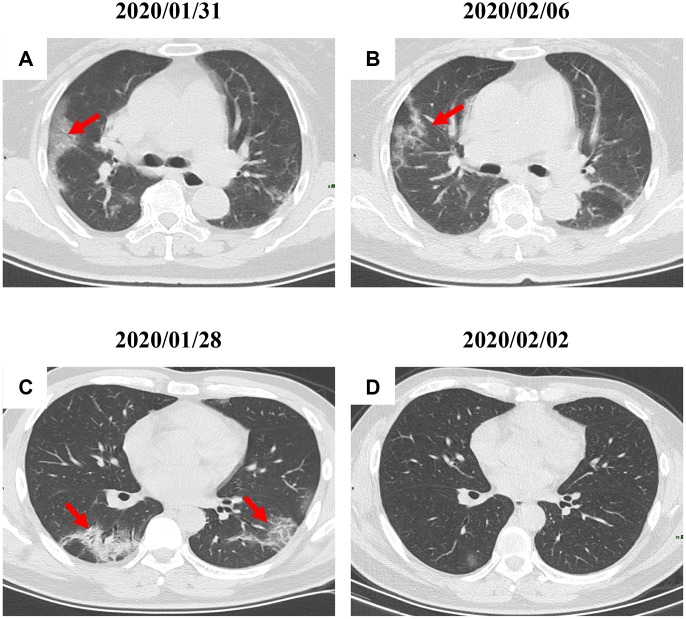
Radiological improvement of two COVID-19 pneumonia patients. (A, B) Patch lesions were absorbed and changed into reticular spline ones (a 31-year-old female patient); (C–D) Significant improvement of CT sign was achieved in a 22-year-old male patient. Typical lesions were marked with red arrows.
